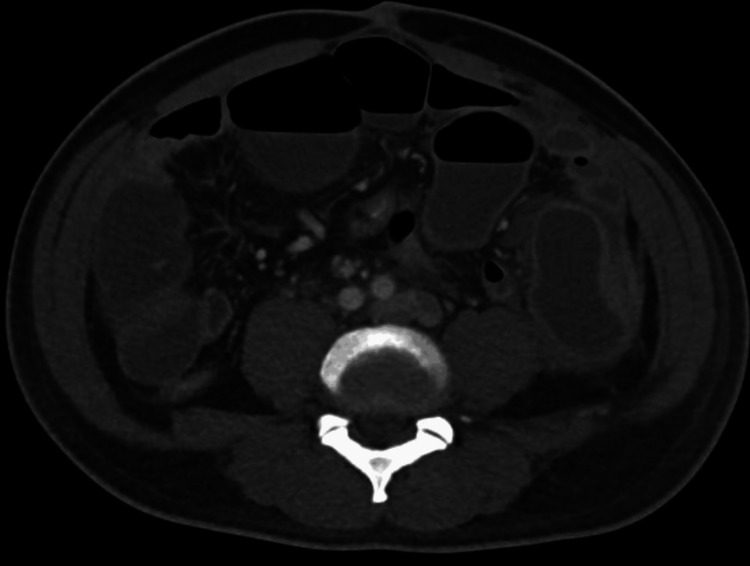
Axial contrast-enhanced CT image demonstrating marked dilation of proximal small-bowel loops with relative decompression of distal small bowel in the right lower quadrant and left paracolic gutter, findings consistent with a high-grade small bowel obstruction.
